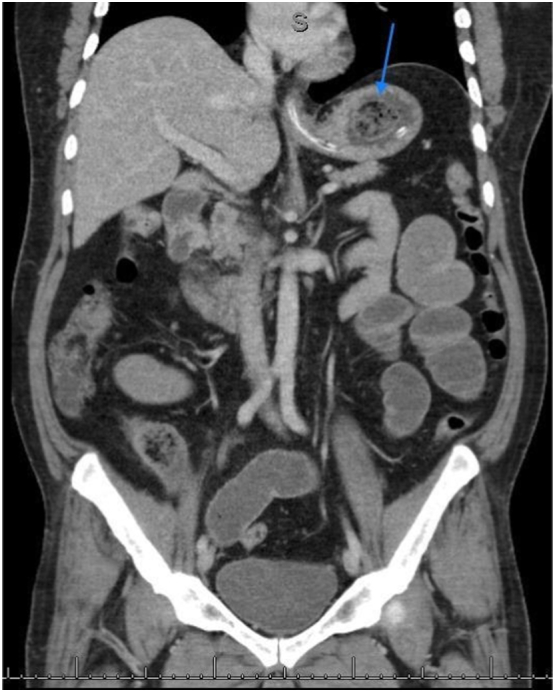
A sagittal plane of CT abdomen showing a large intra-gastric mass, pointed by the blue arrow.
